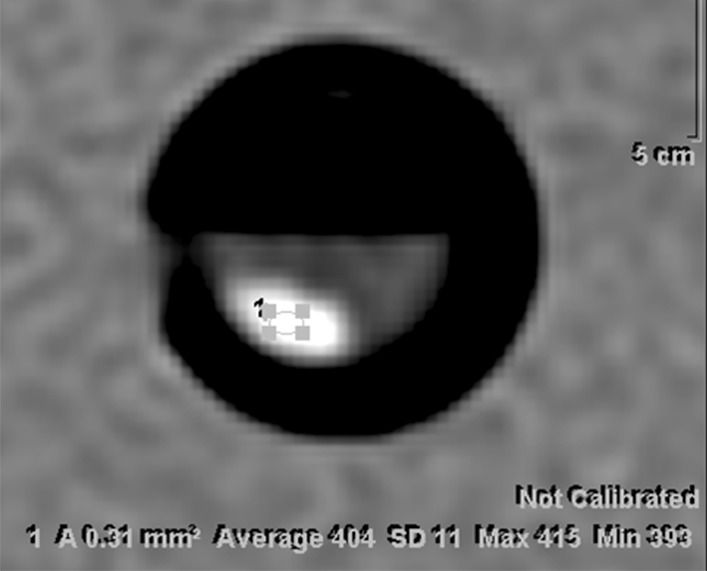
In vitro CT of xanthine urinary stones: magnified axial CT image showing measurement of CT number utilizing region of interest sampling
